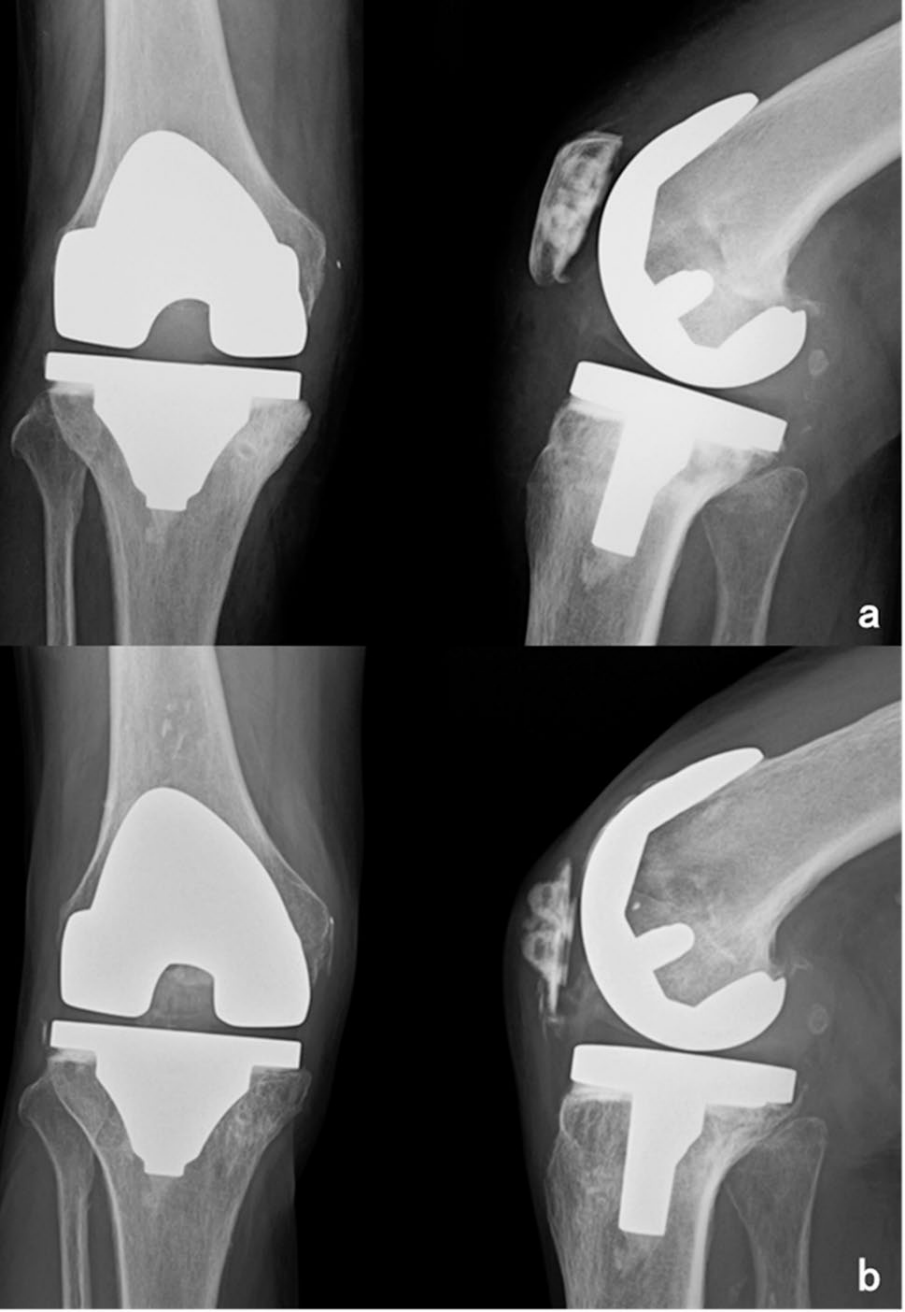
Radiographs of the right knee. (a) Radiograph obtained immediately after total knee arthroplasty, (TKA) using the Scorpio NRG PS implant, (Stryker, Marwah, NJ, USA), (b) Radiograph obtained after the patient presented with knee pain. Fourteen years post-surgically, no loosening was observed
